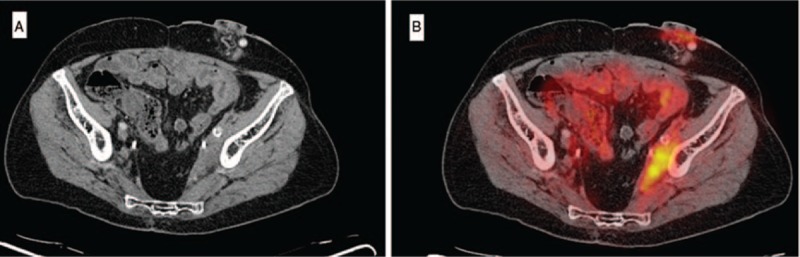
(A) CT pelvis showing a side wall soft tissue mass extending inferiorly and encasing the left iliac vessels and ureter. (B) Associated intense FDG avidity on concurrent PET imaging.
